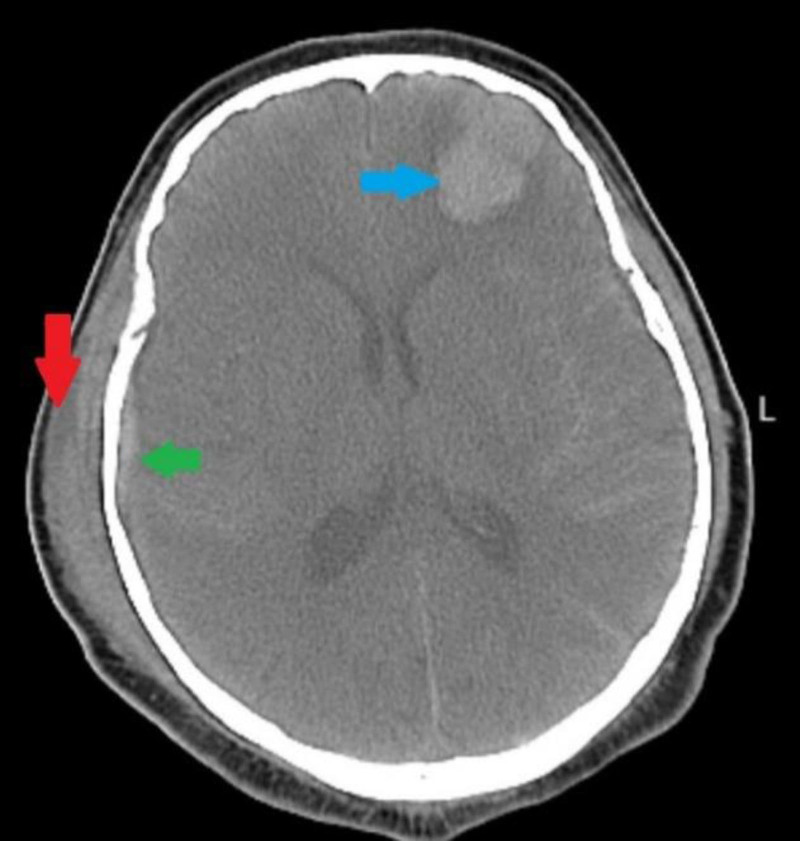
An axial non-contrast head CT image shows a right parietal epidural hematoma measuring approximately 7 cm × 2.5 cm (red arrow), accompanied by an underlying linear, minimally displaced skull fracture and pneumocephalus (green arrow). Bilateral diffuse subarachnoid hemorrhage is evident, alongside a small left tentorial subdural hematoma measuring 0.5 cm × 4 cm (blue arrow). Notably, there is no observable midline shift, indicating no significant mass effect. CT = computed tomography.
